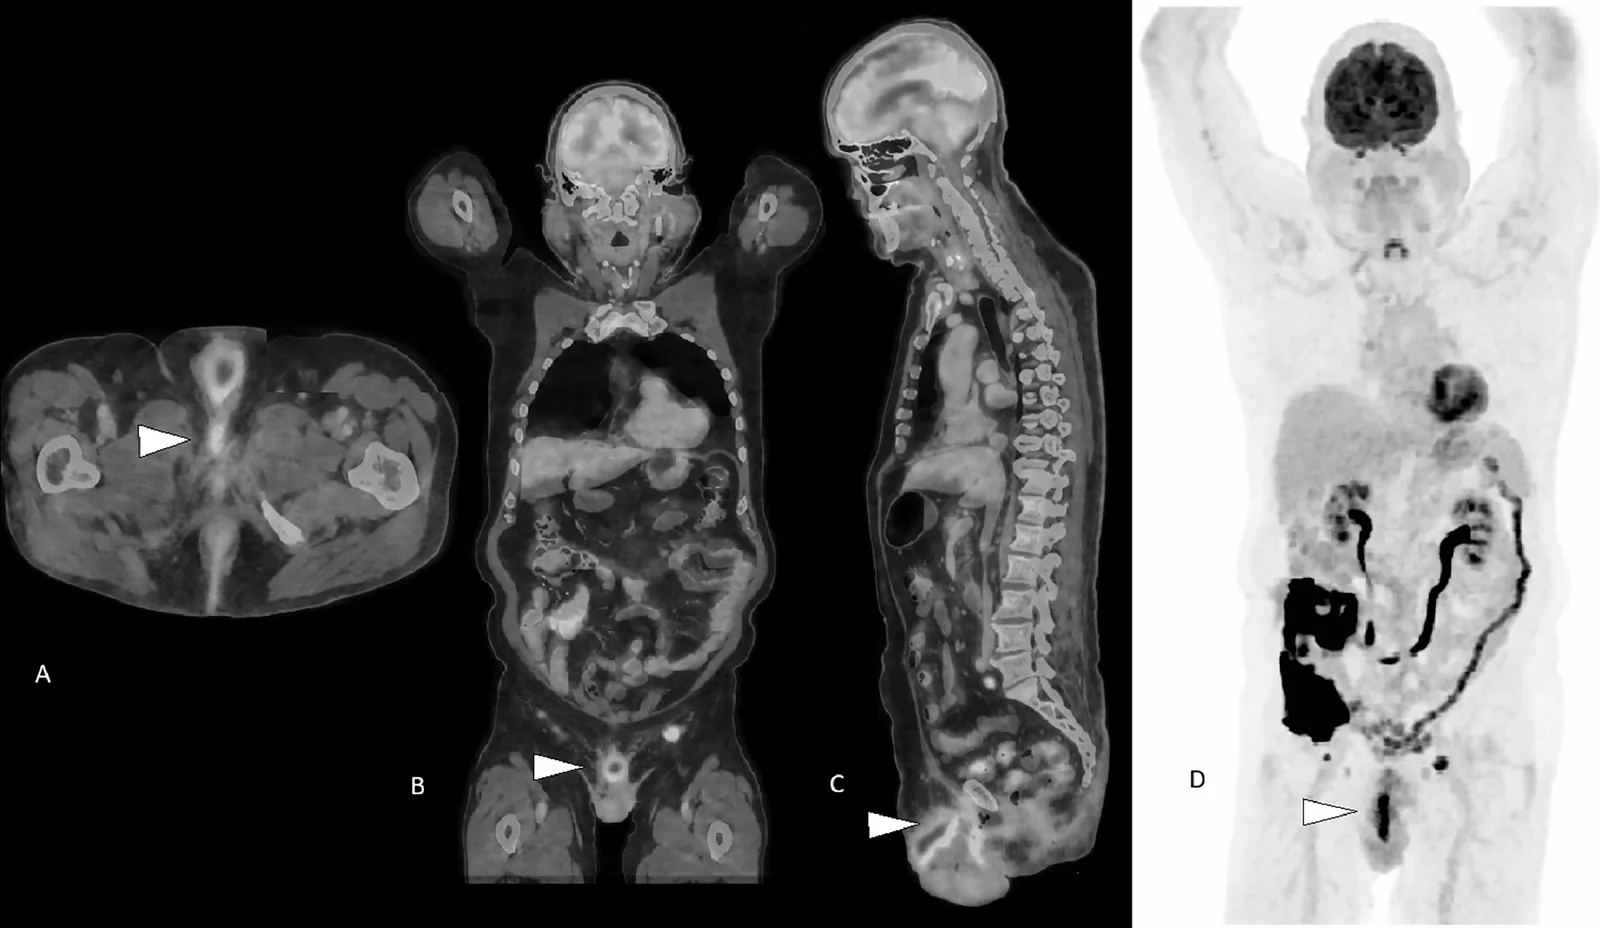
A) Axial, B) Coronal and C) Sagittal fused ^18^F–FDG PET/CT images, together with D) maximum intensity projection (MIP) PET image, obtained 6 weeks following urethrectomy, demonstrated persistent intense FDG uptake adjacent to the inferior margin of a postoperative fluid collection within the urethrectomy bed (arrowheads). The findings favoured post operative inflammatory activity, confirmed by repeat biopsy showing granulation tissue and chronic inflammation. ^18^F–FDG PET/CT also demonstrated the development of FDG-avid bilateral inguinal lymph nodes, more pronounced on the left, consistent with regional nodal metastatic progression. No distant FDG-avid metastatic disease was identified.
